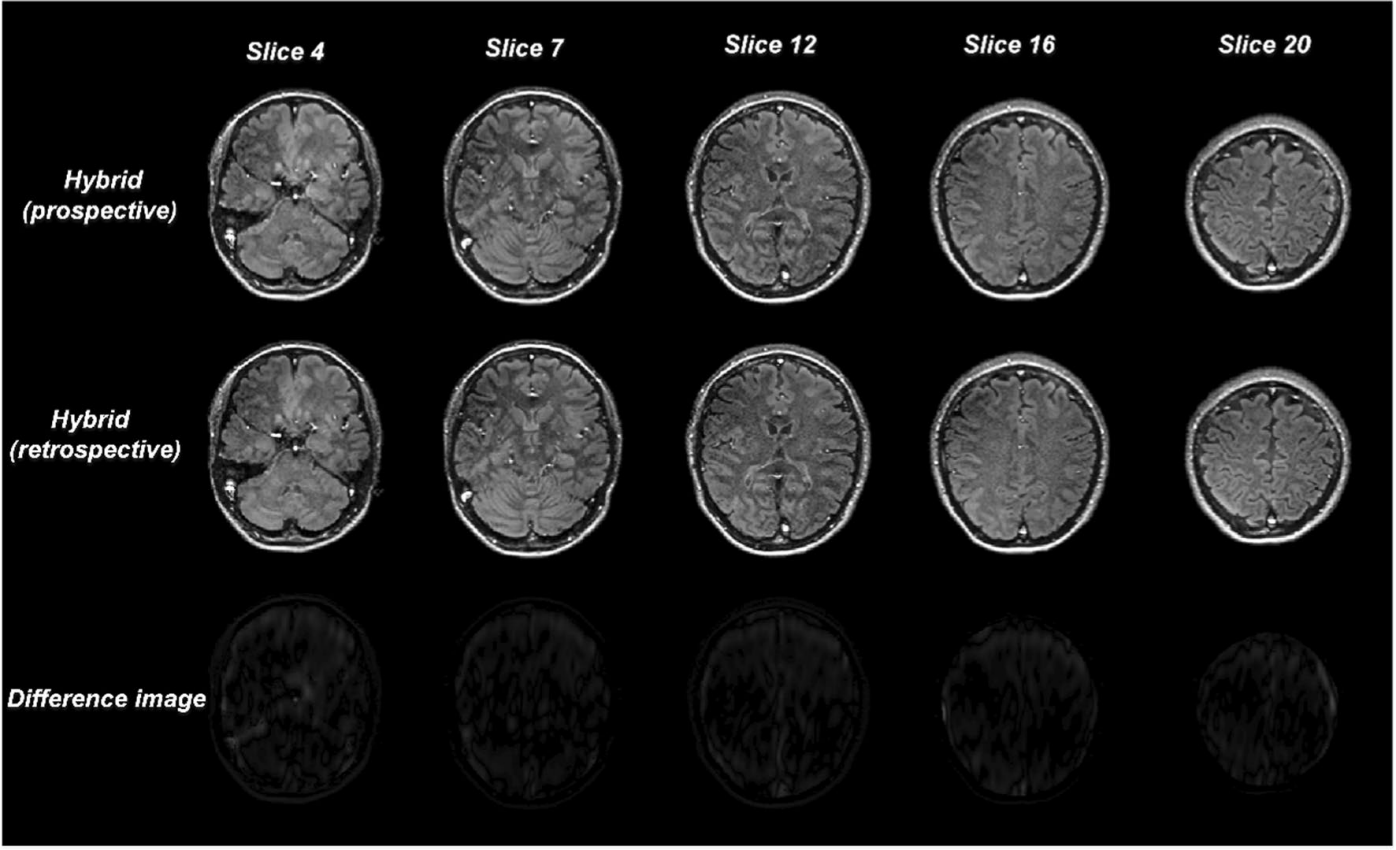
Comparison of prospective and retrospective hybrid FLAIR images from different slices of a healthy volunteer’s brain when applying a 12.5% under sampling in the conventional FLAIR acquisition. The two approaches lead to practically the same result
